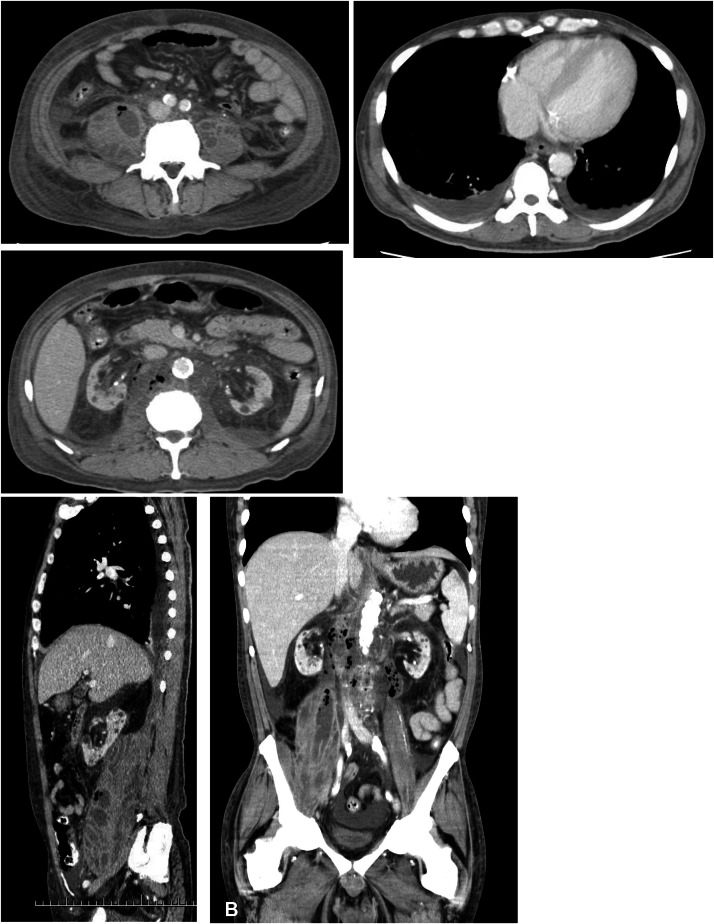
(A) Plain CT scan from 3 d prior shows edematous changes in the retroperitoneum and increased density of the perirenal adipose tissue. A 3-cm abscess is identified within the right psoas muscle (arrow). (B) Contrast-enhanced CT scan at admission shows severe right-sided predominated abscesses in the iliopsoas muscles, an abscess with pneumoperitoneum in the para-aortic region, further increased density of the perirenal fat, additional abscess formation, and right empyema. No intestinal perforation is observed.
